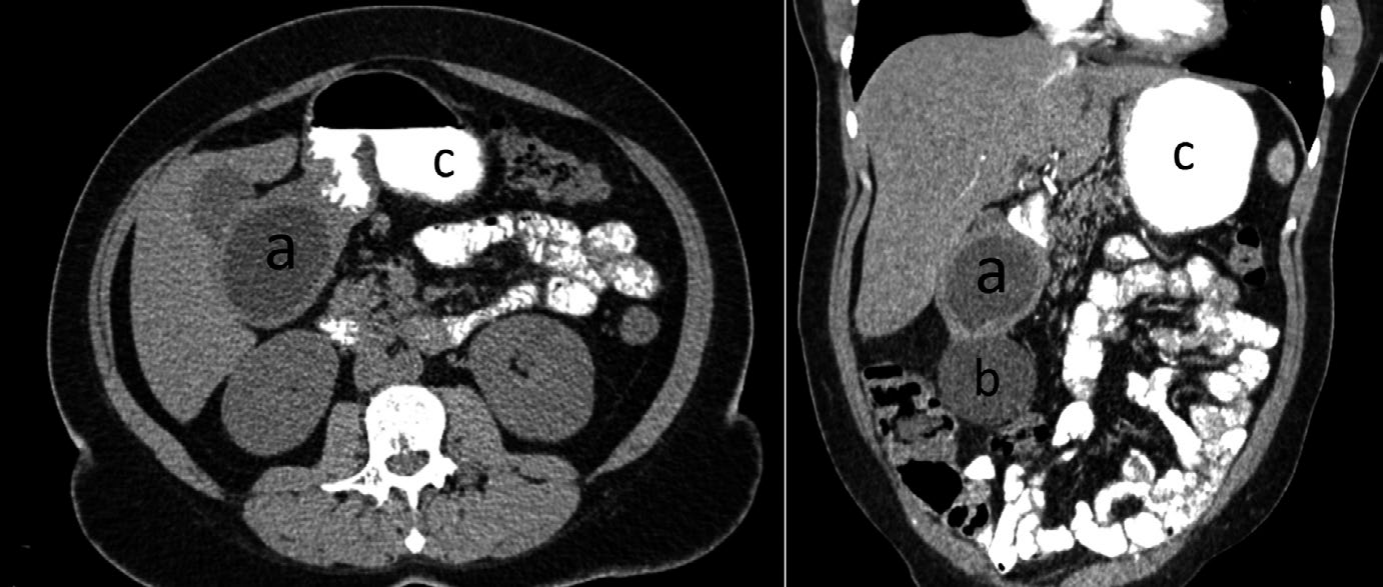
CT scan images showing the two cysts developed in the antro‐pyloric region of the stomach without communicating with its lumen
